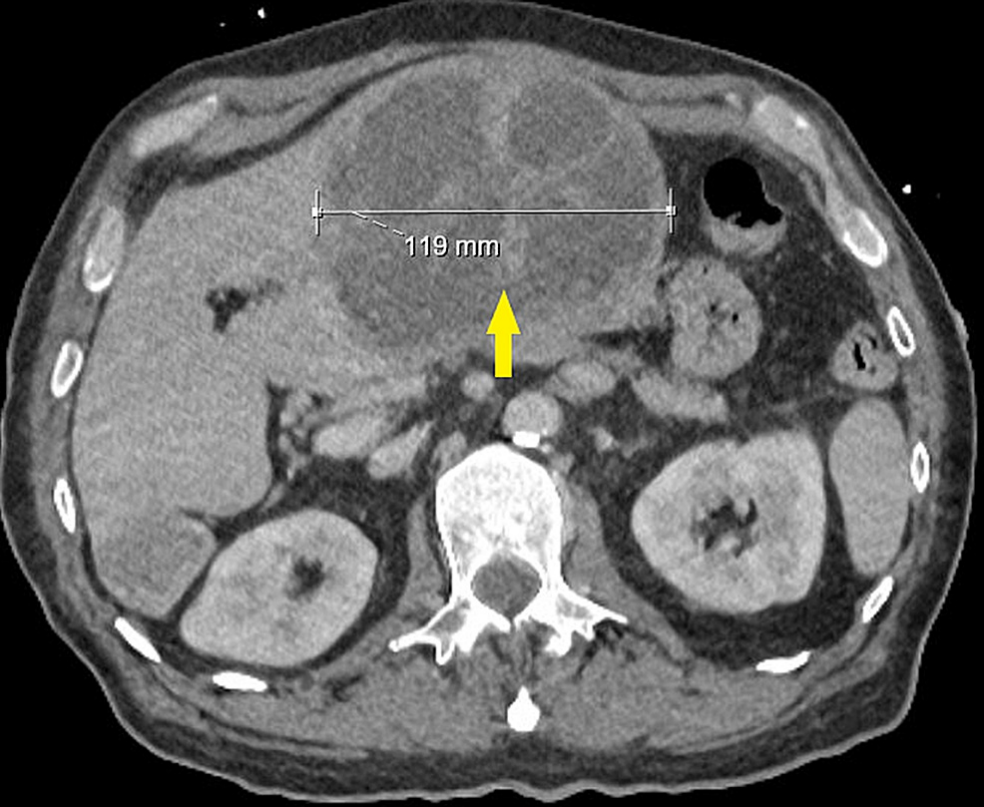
Computed tomography of abdomen showing large multiloculated liver abscess
